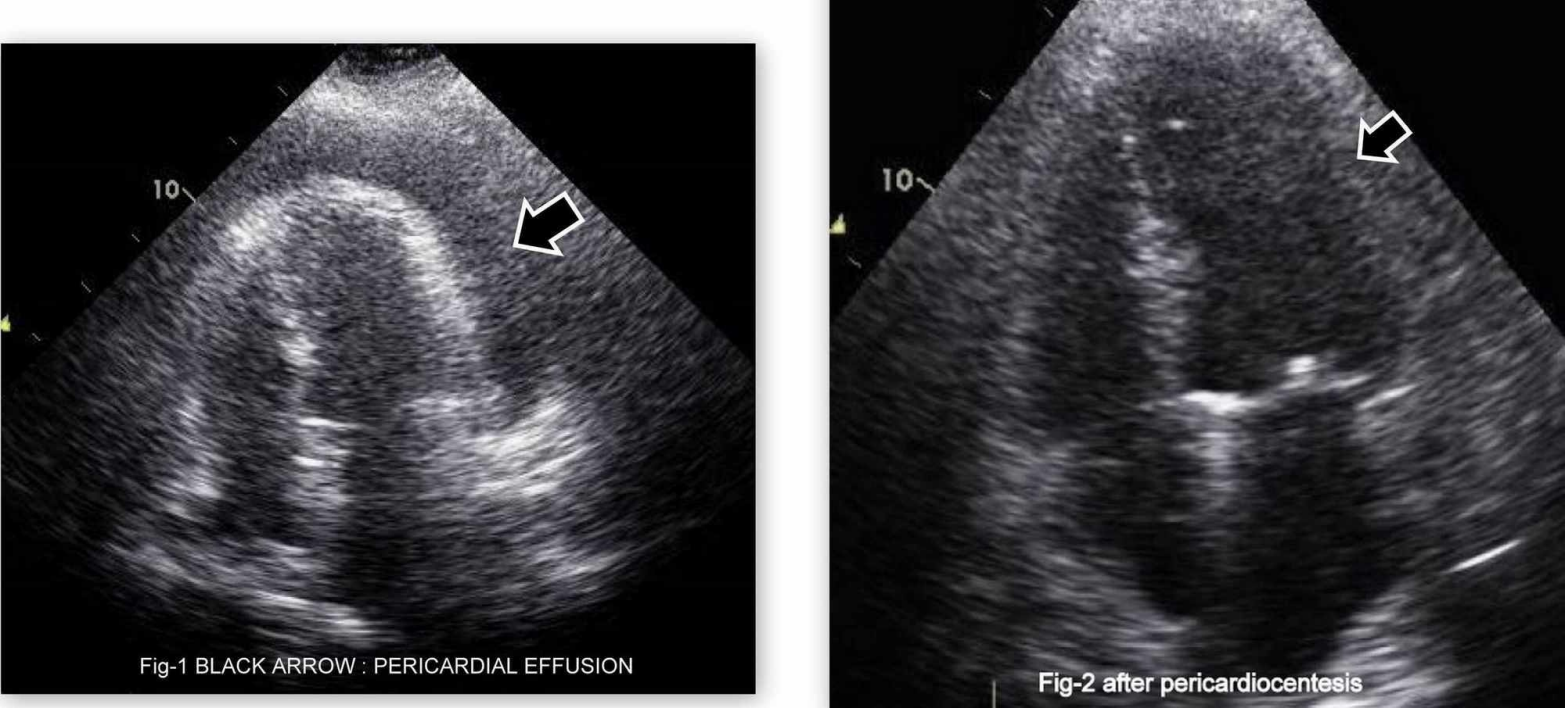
Before (left side) and after (right side) pericardiocentesis echocardiography of the patient
